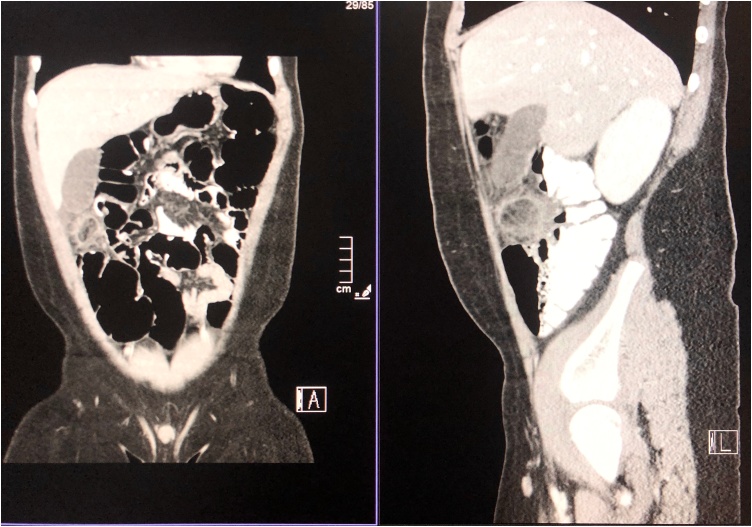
Abdominal CT scan with contrast showing inflammatory stranding and free fluid in the pelvis.
